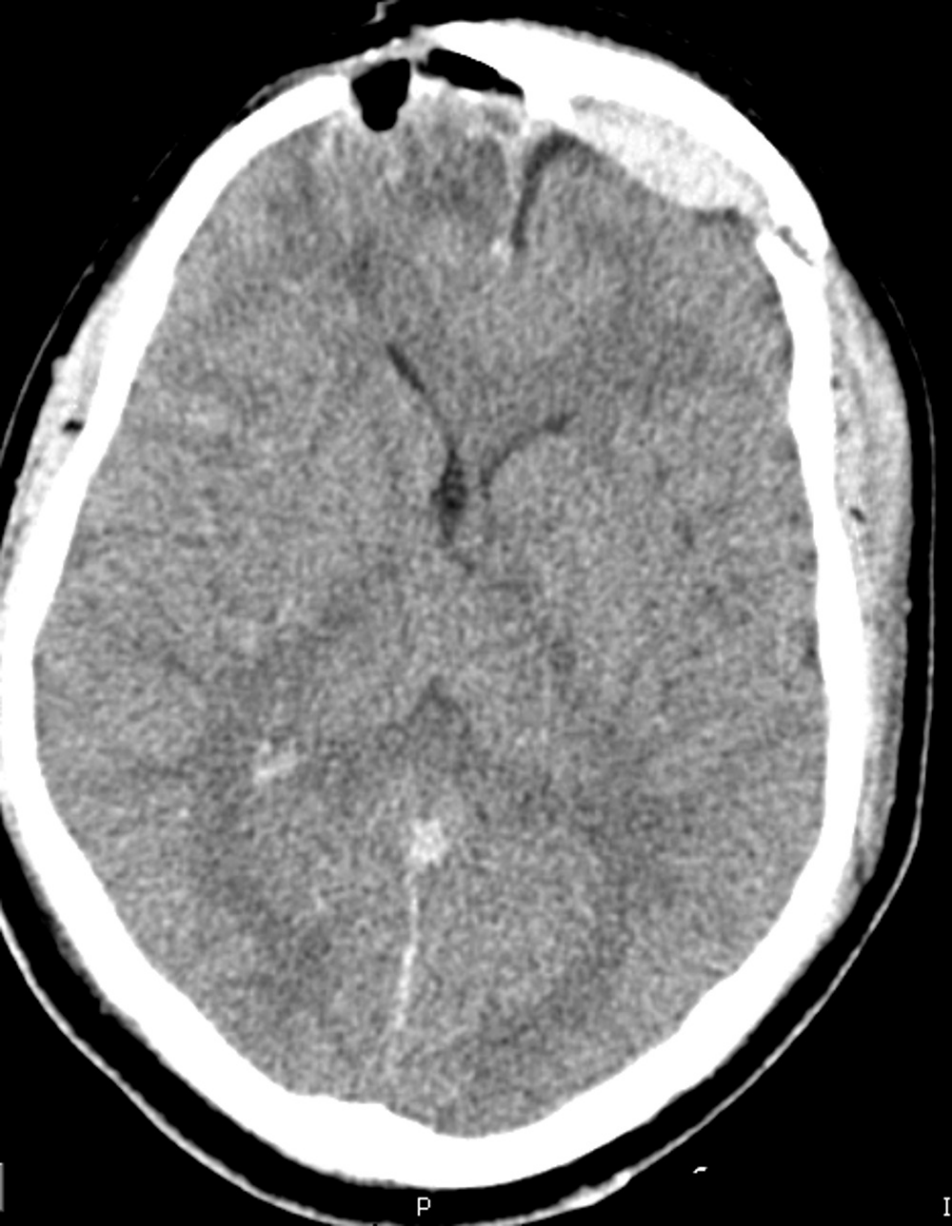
Postoperative CT head showing elevation of fracture fragments
